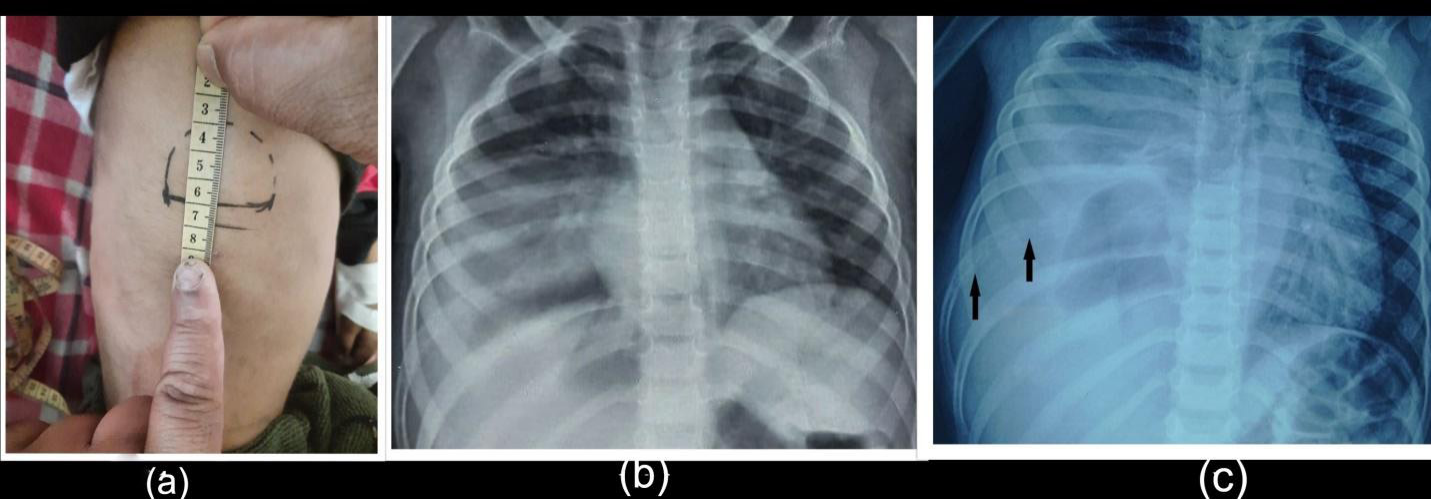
(a) Swelling over right lateral chest wall; (b) X-ray one month back (at initial presentation) to local hospital; (c) at the time of visit to our hospital.
